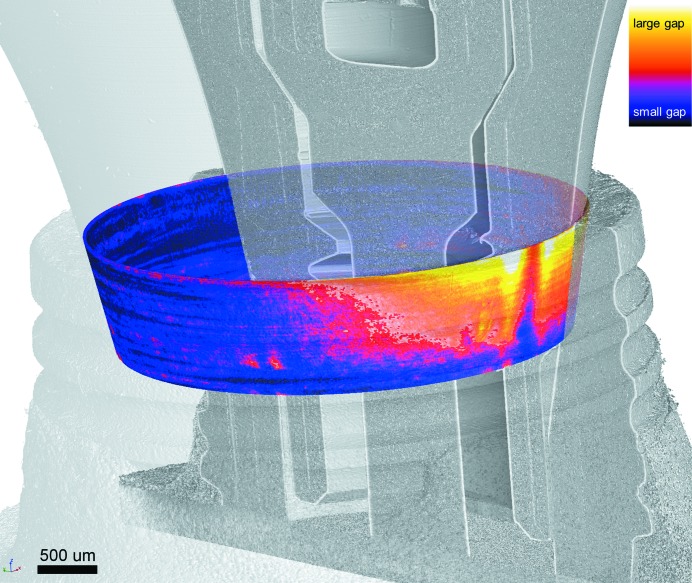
Exemplary three-dimensional visualization of an IAC microgap map (coloured) superimposed on the CT scan of the dental implant, pointing out the orientation of the latter. Here the implant is a NobelActive (Nobel Biocare, Switzerland) under 333 N cyclic load. The IAC map is calculated according to Zabler *et al.* (2012 ▸); the look-up table is comparable with Fig. 6 ▸.
